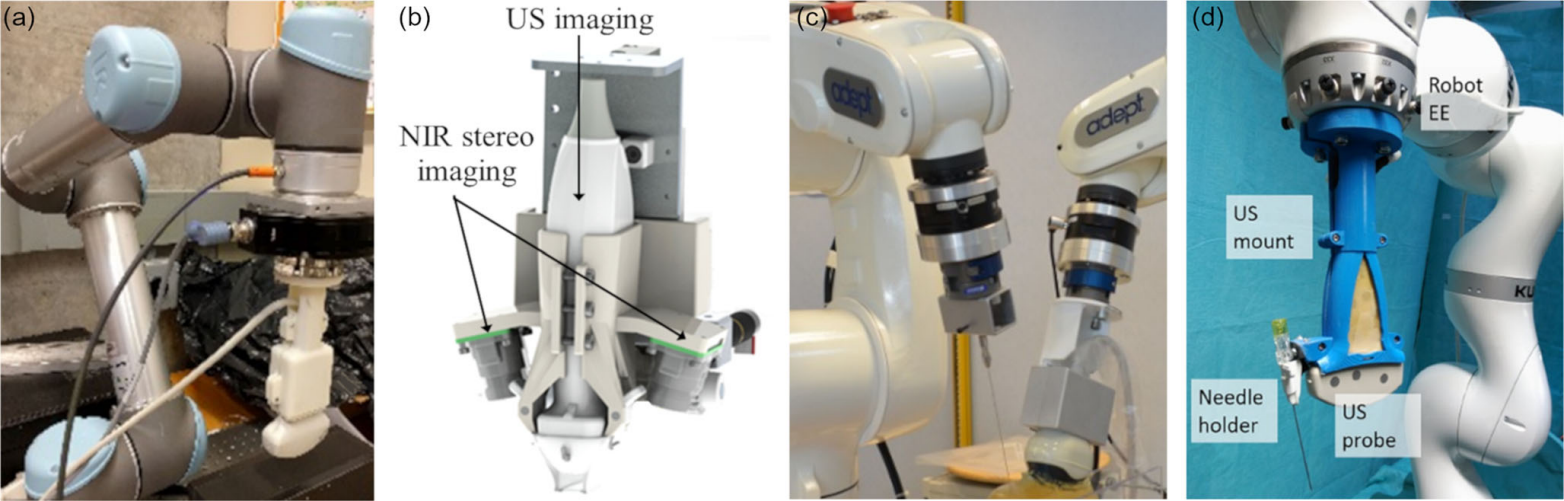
Overview of system components for collaborative assisting robotic ultrasound systems. a The *STRATUS* system including a *UR5* robot and an ultrasound probe interconnected by a six DOF force-torque sensor (copyright © [2016] IEEE. Reprinted with permission from [35]). b Near infrared imaging sensors combined with an ultrasound probe for bimodal vessel imaging in the forearm to guide venipuncture (reproduced from [37•] with permission from Springer Nature). c Setup for a flexible needle steering system of two *Viper s650* robots (Adept) with needle holder and ultrasound probe (copyright © [2015] IEEE. Reprinted with permission from [38]). d
